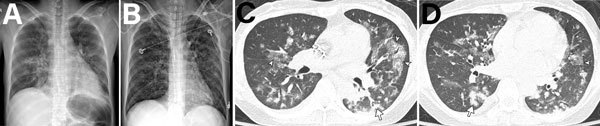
Imaging of 38-year-old woman in South Korea who underwent liver transplantation 2 months earlier, followed by immunosuppressant therapy, who visited the emergency department because of dyspnea, chills, and fever. A) Initial chest radiograph showing bilateral peribronchial infiltration in the central areas of both lungs and minimal pleural effusion. B) Five days later, the patient was admitted to the intensive care unit and underwent intubation because of progressive dyspnea. Radiograph showed extensive bilateral peribronchial nodular infiltrates. C, D) Computed tomography performed on the same day as in panel B showing multifocal peribronchial nodular consolidation (arrows), ground-glass opacities (arrowheads), and irregular small nodules, along with small amounts of bilateral pleural effusion. The patient was given ribavirin and prophylactic antimicrobial drugs and recovered after 10 days in the intensive care unit.
